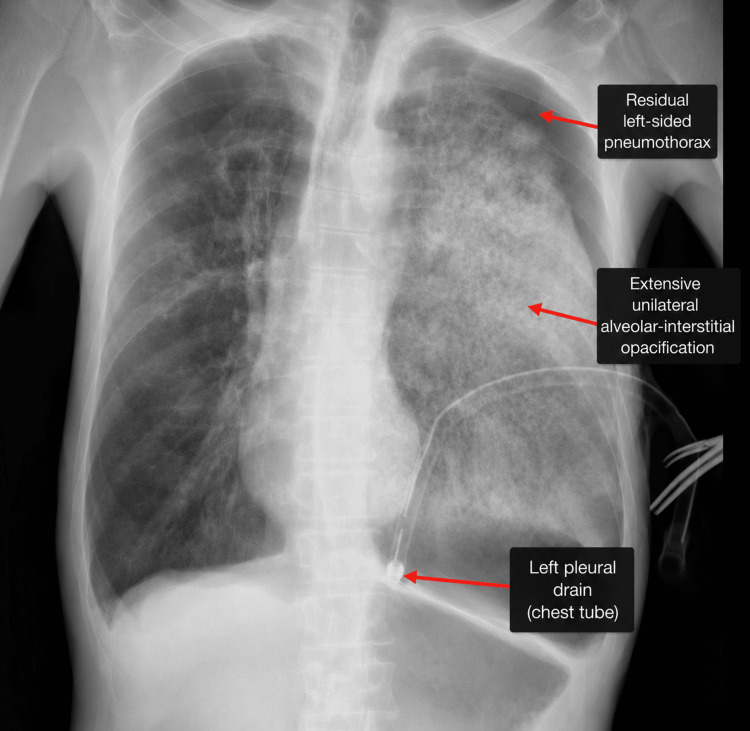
Chest radiograph demonstrating unilateral re-expansion pulmonary edema following pleural drainage Follow-up chest radiograph obtained after pleural drainage demonstrated residual left-sided pneumothorax (arrow); extensive unilateral alveolar-interstitial opacification involving the re-expanded left lung, consistent with presumed re-expansion pulmonary edema (arrow); and a left pleural drain (arrow). The right lung remains relatively spared apart from chronic emphysematous changes.
